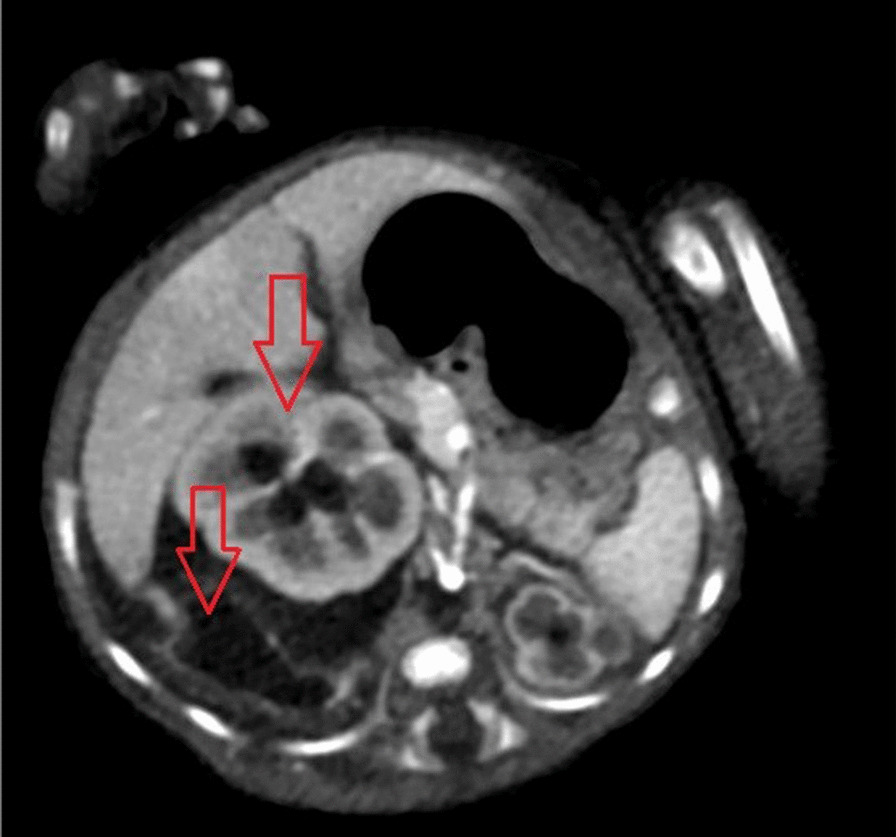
Abdomen CT shows right-side hydronephrosis with fluid leakage retroperitoneum (Arrows)
